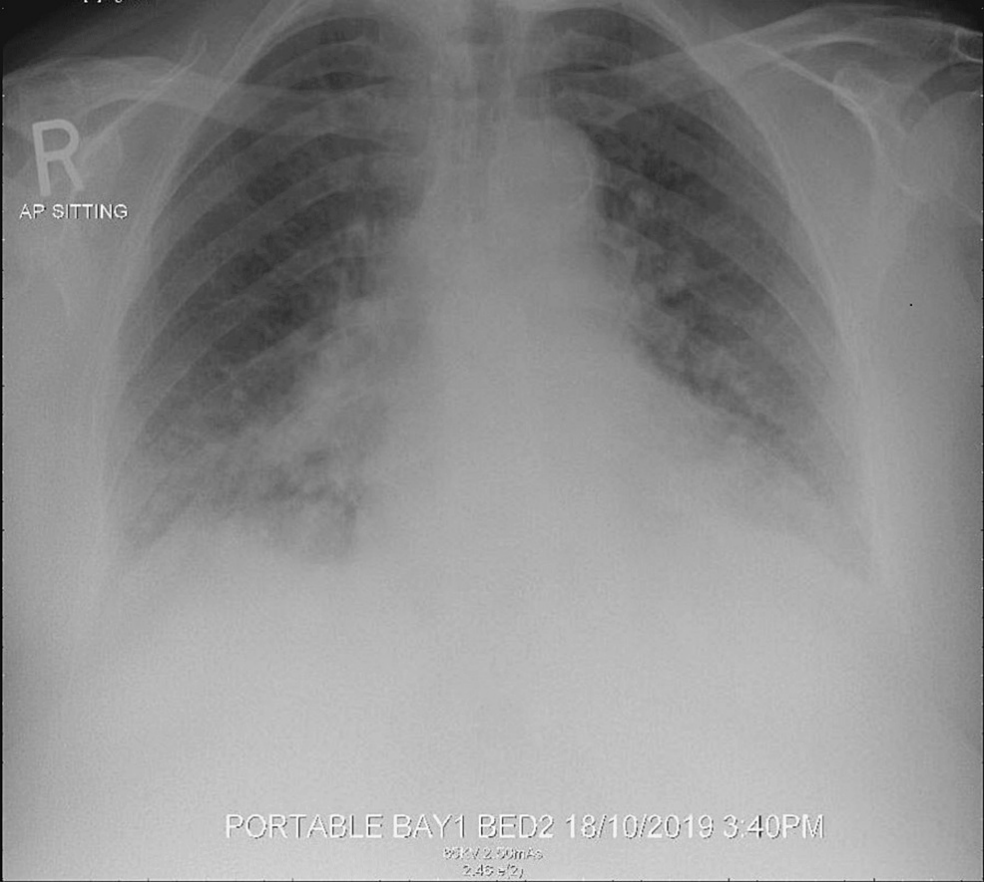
Chest x-ray showing pulmonary edema on the patient’s second admission
